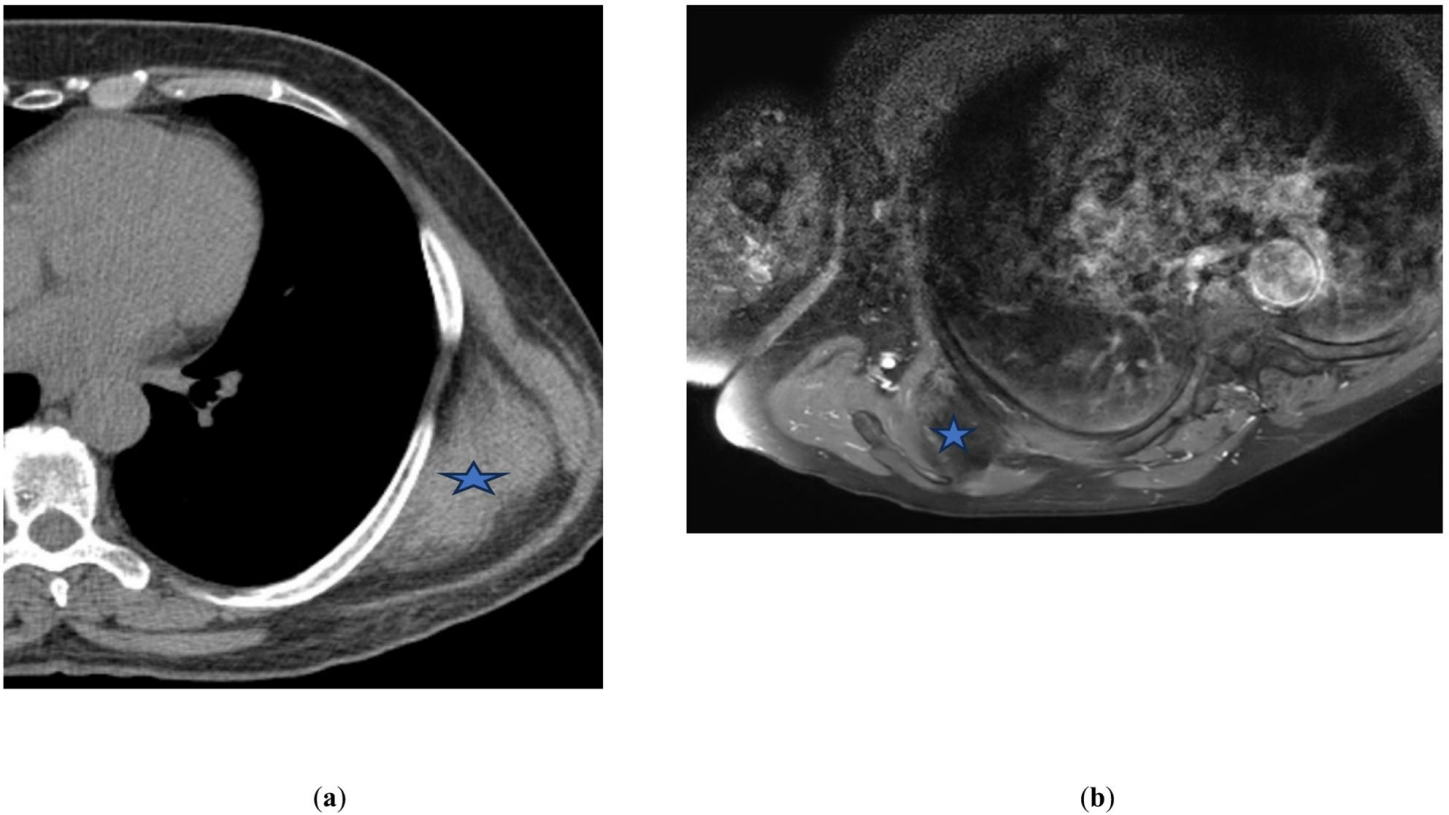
Radiological appearance of elastofibroma dorsi. a Axial computed tomography scan demonstrating a poorly circumscribed soft tissue mass in the subscapular region with alternating areas of soft tissue and fat attenuation (blue asterisk). b Axial T1-weighted magnetic resonance image showing a heterogeneous lesion with characteristic interlacing fibrous and fatty components, consistent with elastofibroma dorsi (blue asterisk)
